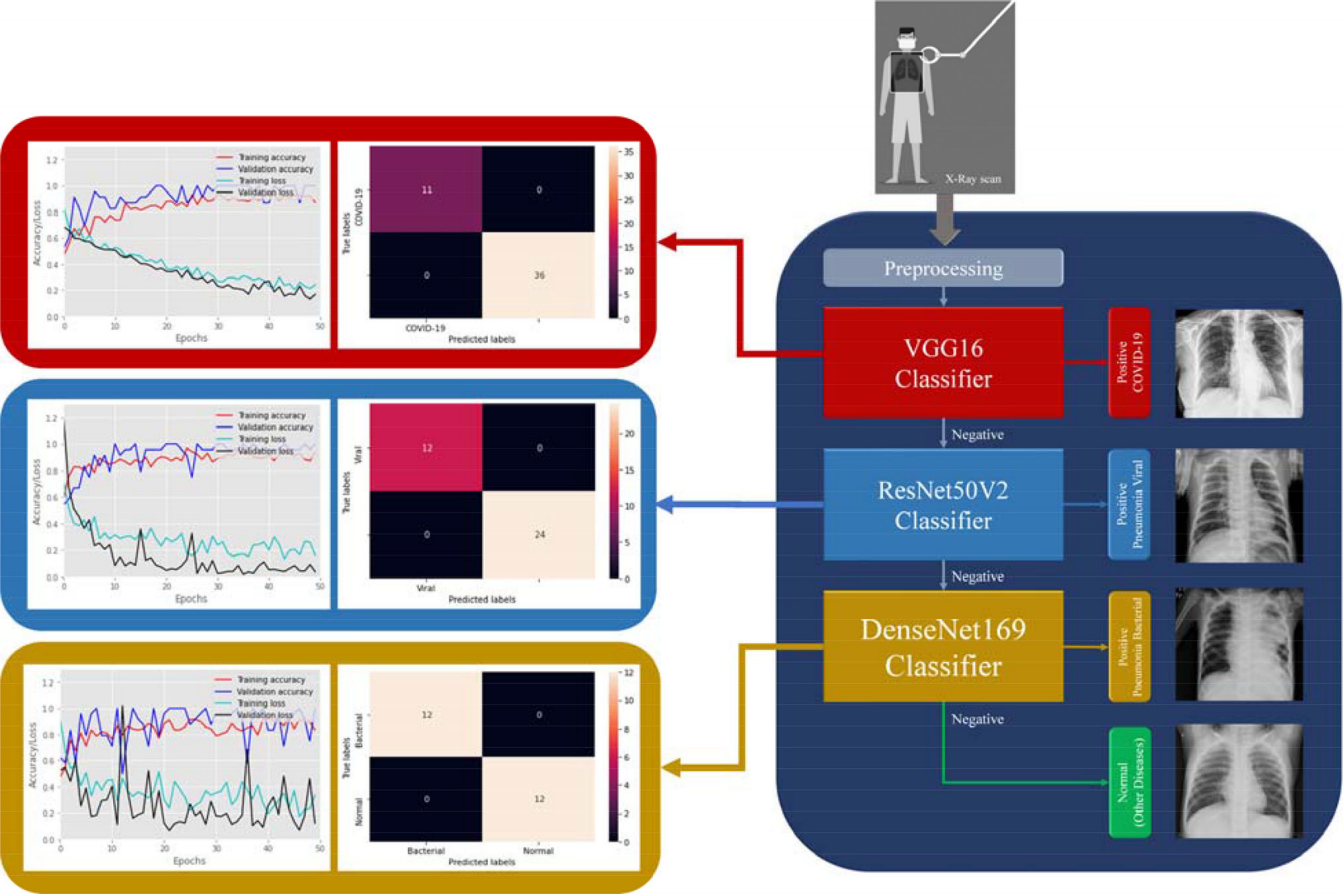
Final recommended deep X-ray image classifiers for detecting COVID-19, viral and bacterial pneumonia diseases using VGG16, ReseNet50V2 and DenseNet169 models, respectively (right). The corresponding accuracy and loss curves with resulted confusion matrix are linked to each image classifier (left)
